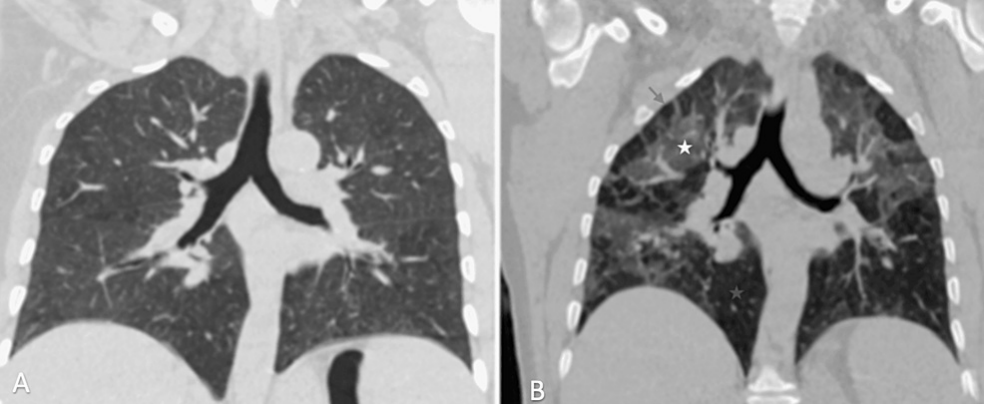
Chest CT scan in the parenchymal window showing an appearance of three densities. Chest CT scan in the axial window in inspiration (A) and forced expiration (B) showing healthy parenchyma, air trapping, and increased ground glass.
